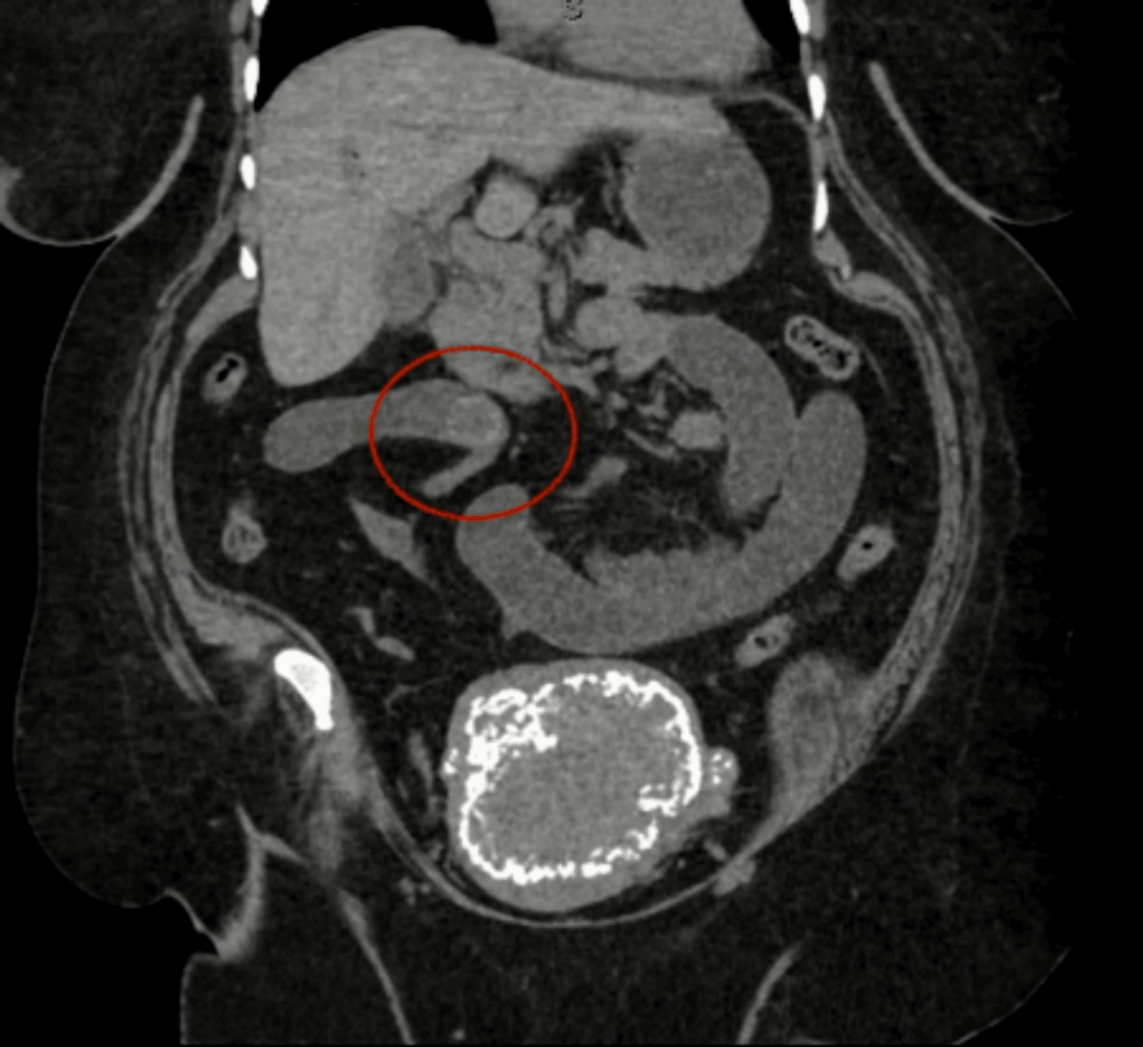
CT demonstrating a stone in the small bowel (circled in red)
